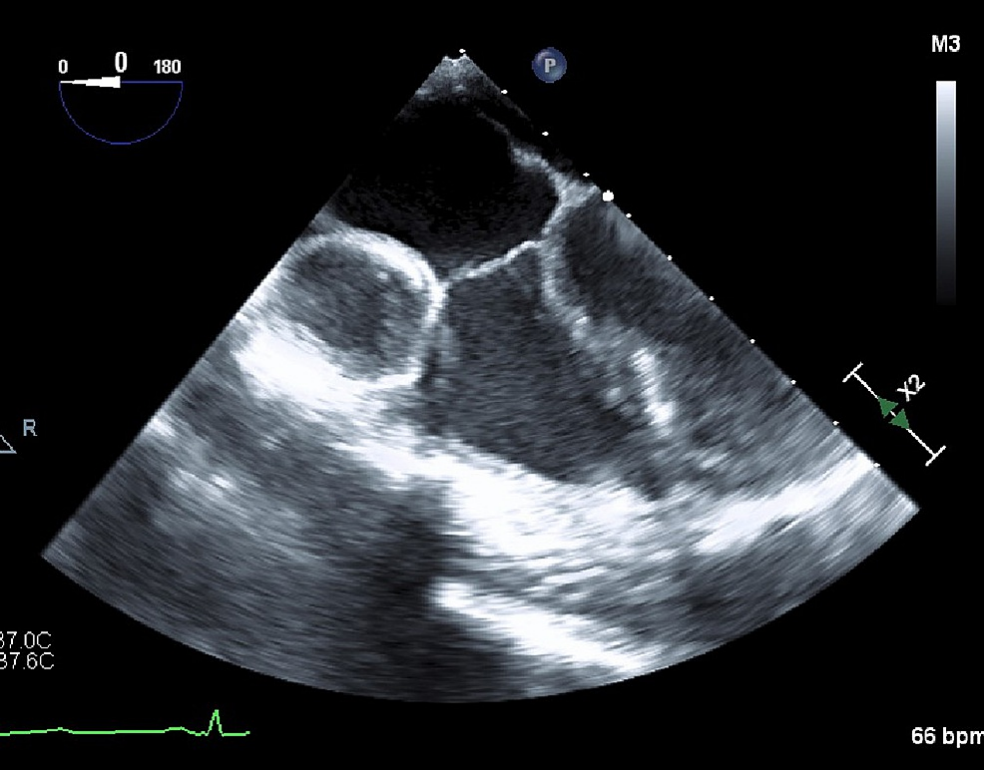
Mid-esophageal four-chamber view from intraoperative TTE demonstrating large aneurysm of right coronary artery with thrombus burden TTE, Transthoracic echocardiogram.
